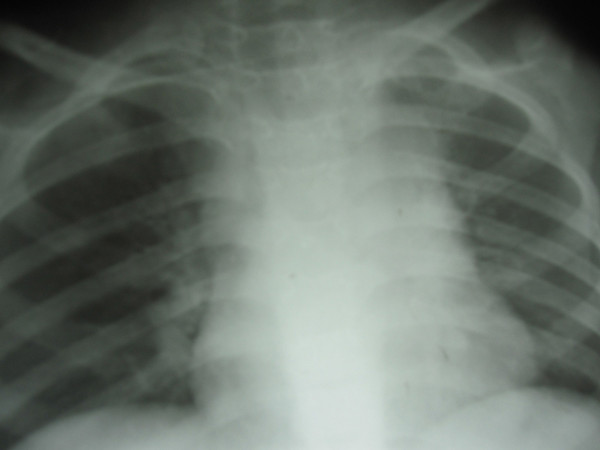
Chest X-ray showing mediastinal dilation at first presentation.
